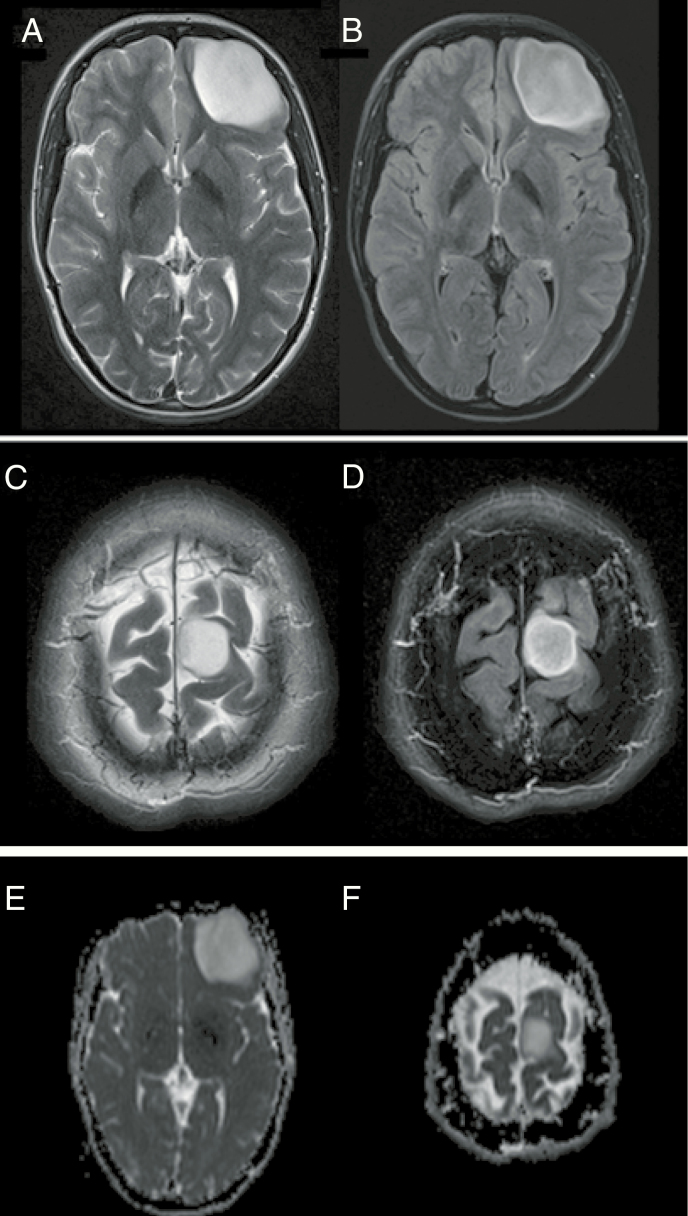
Two cases with the presence of a T2/FLAIR-mismatch sign and their corresponding ADC image (E and F). (A and B) IDH-mutant 1p/19q non-codeleted anaplastic astrocytoma (WHO °III) in a 31-year-old man and (C and D) IDH-mutant 1p/19q non-codeleted anaplastic astrocytoma (WHO °III) in a 56-year-old woman. Both cases show tumors located in the left frontal lobe with a homogeneously hyperintense signal on T2-w images (left side), coupled with a homogeneously hypointense signal on FLAIR images except for a small hyperintense peripheral rim (right side).
